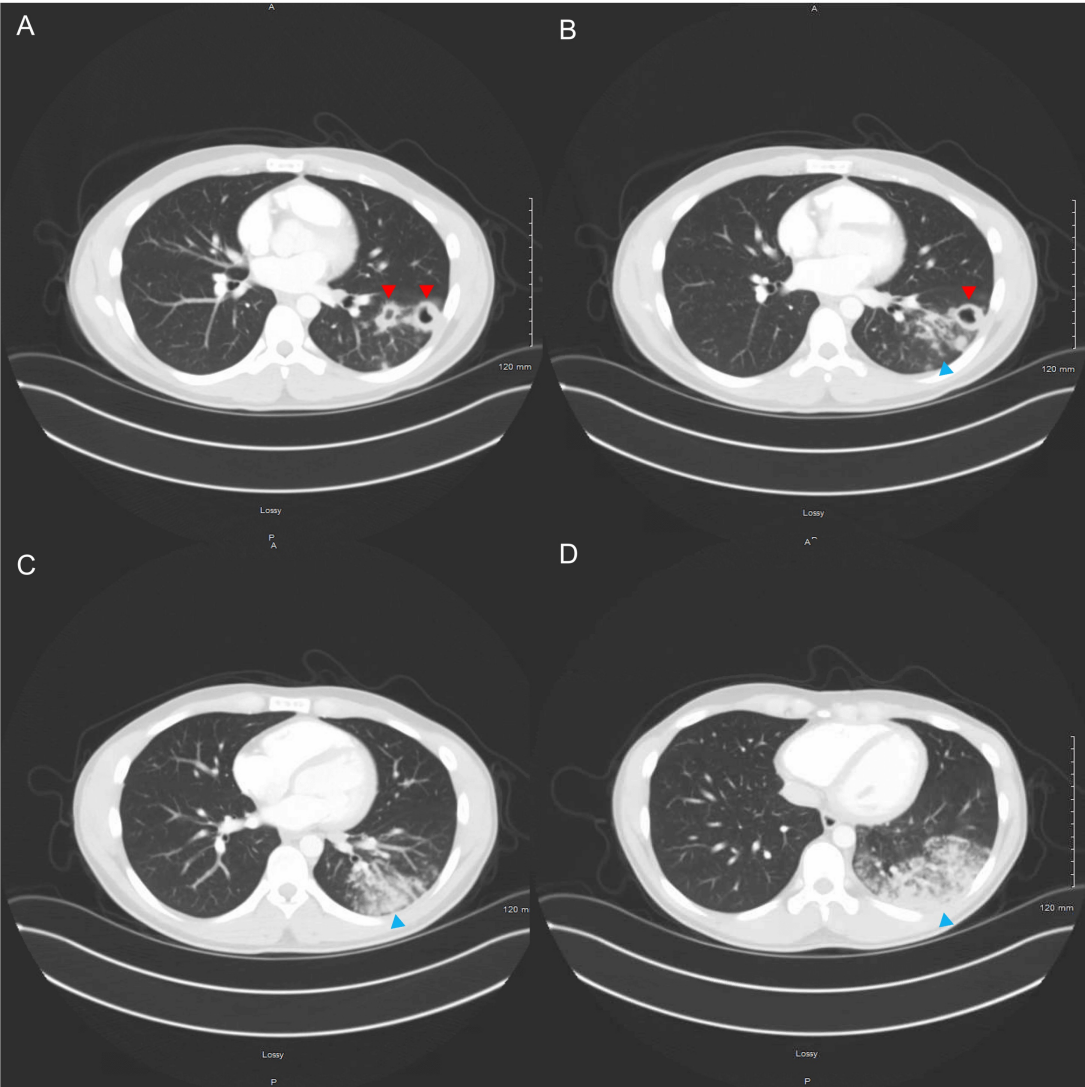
Axial CT Scan of the Chest With IV Contrast; Lung Window (Panels A–D, Cranial to Caudal) Cluster nodular and ground-glass density infiltrates with air bronchogram (blue arrowheads) and two small cavitations in the left lower lobe (red arrowheads). Thin-walled air-filled spaces in the left upper lobe, initially described as pneumatoceles, are visible. However, given the clinical context and eventual diagnosis of tuberculosis, these are favored to represent cavitations secondary to reactivation TB.
